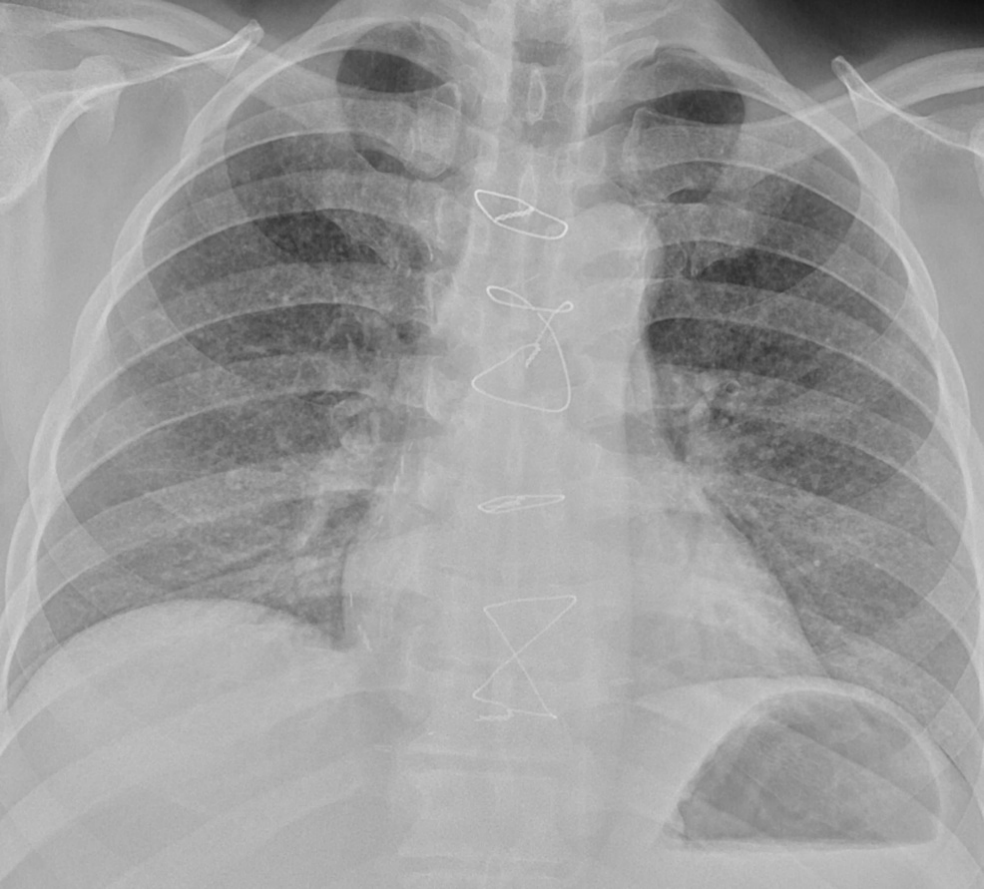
Chest radiograph showing miliary TB features TB: tuberculosis
